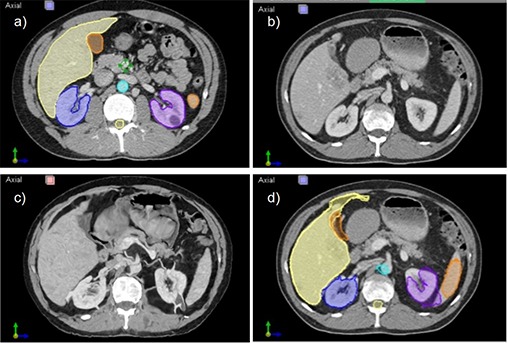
Concept of atlas based segmentation. The template CT dataset with the structures carefully segmented is shown in (a). The aim is to adapt the template dataset to the patient's CT (b) through deformable registration to reproduce organ boundaries. The warped template is shown in (c) and should ideally match (b) in major organs location and shape, with the unusual HU warping outside critical organs normal for interpatient registrations. The atlas segmentation as warped with the deformable registration result to segment the patient's CT is shown in (d).
